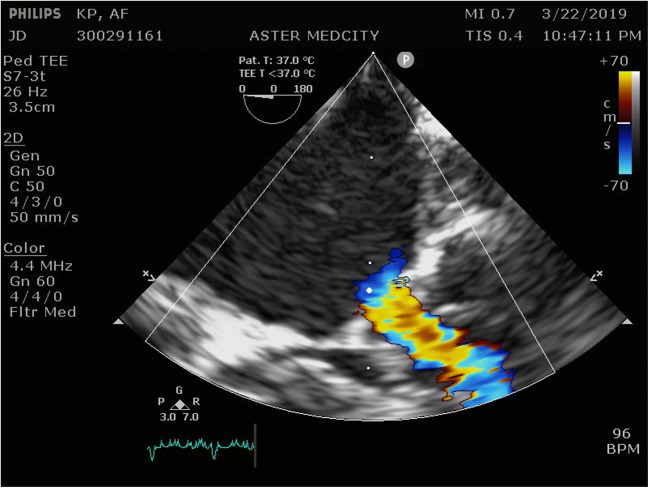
Polar light sign in echocardiogram
